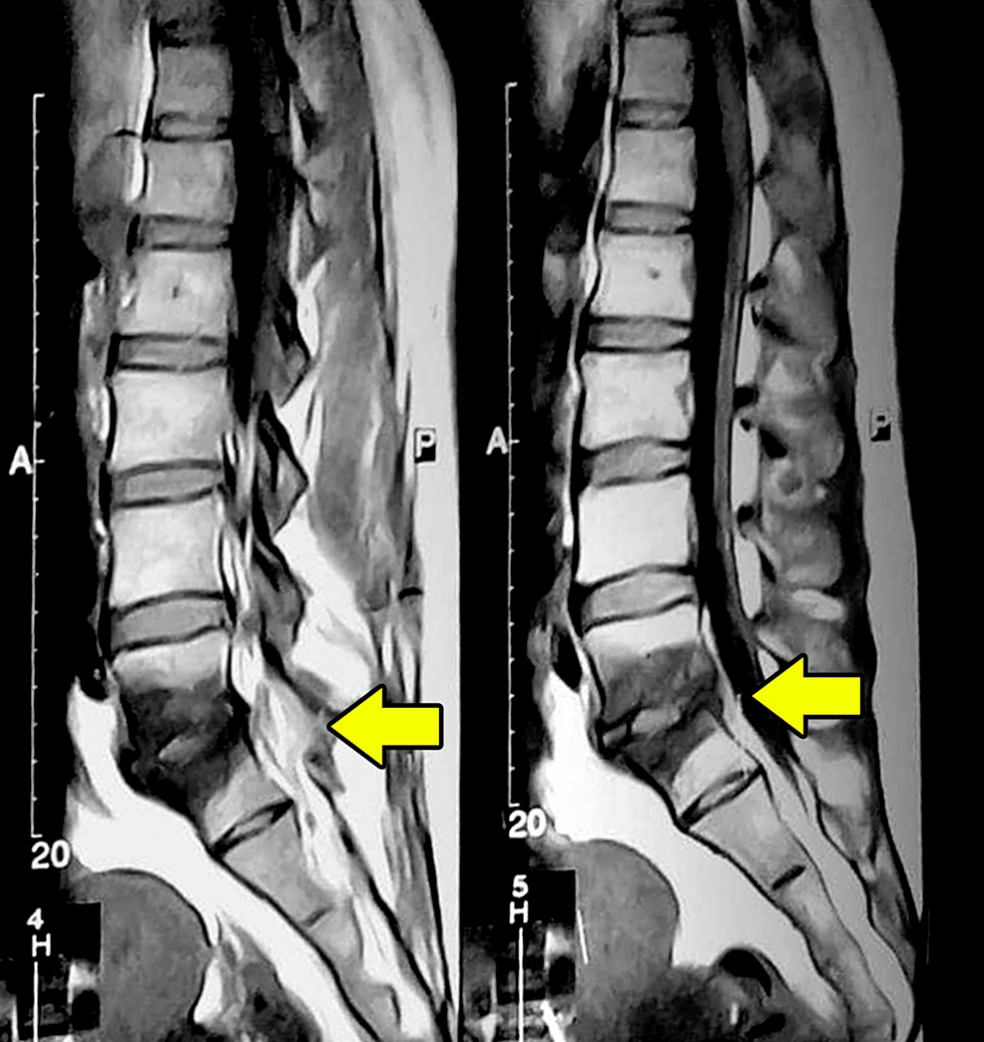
Pre-treatment MRI images. Two sagittal sections of the T1-weighted MRI on initial presentation showing involvement of the L5-S1 disc space and adjacent vertebral bodies with decreased signal intensity. No lesions are seen in the other disc spaces or vertebrae.
